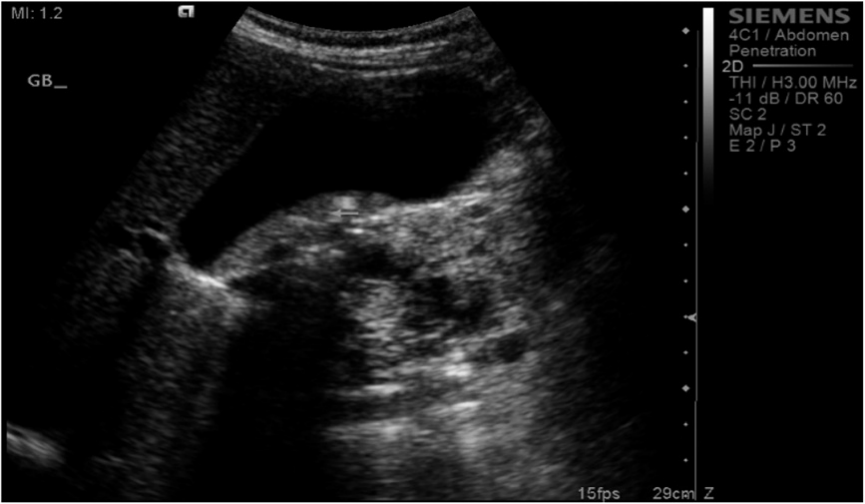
Abdominal ultrasonography showing multiple pancreatic cysts and a dilated biliary system with common bile duct stones.
